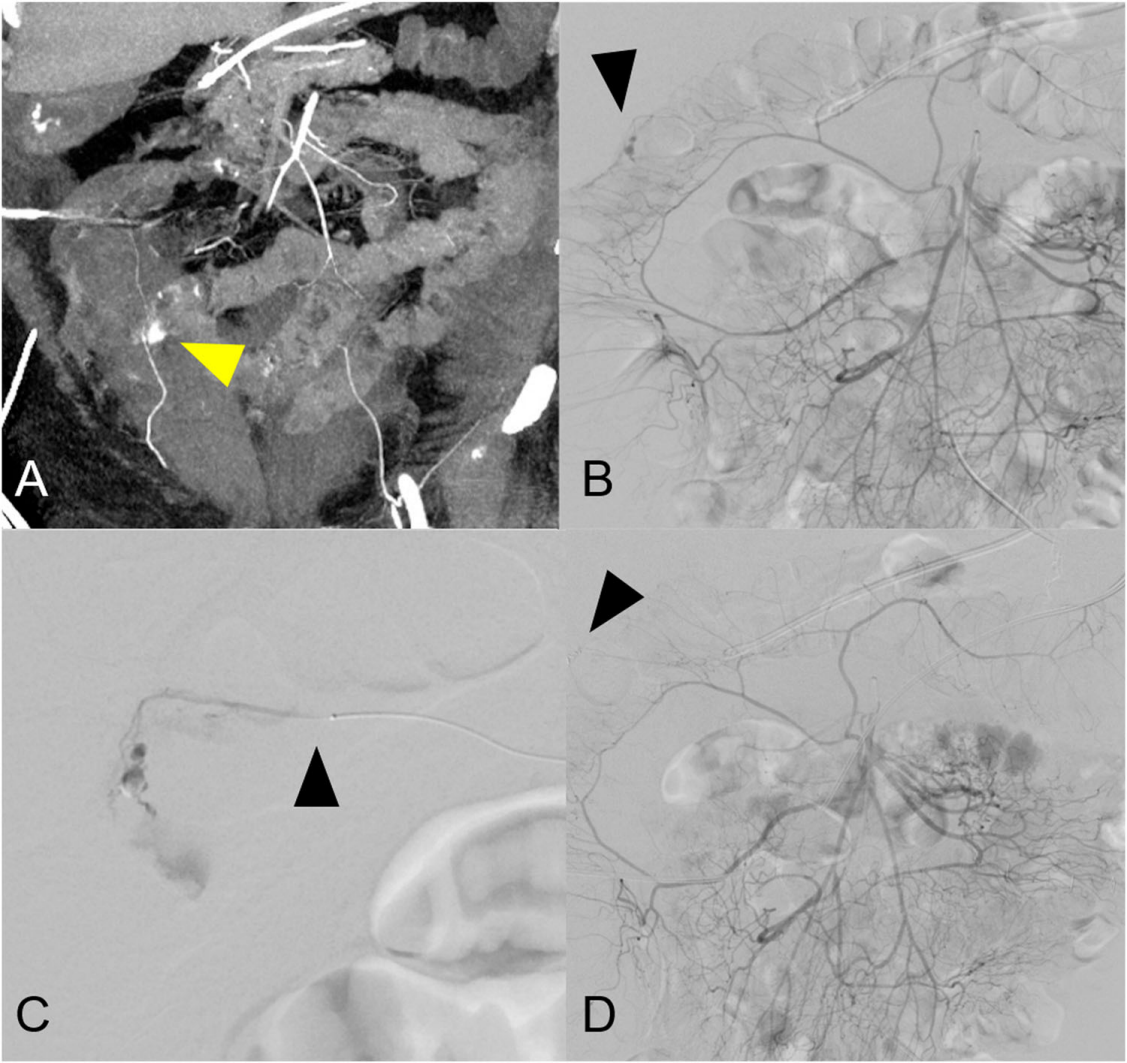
A Contrast-enhanced CT in the arterial phase and coronary slice of a patient with massive rectal bleeding following heart surgery and anticoagulation, where endoscopy revealed no abnormalities. The CT scan identified active arterial bleeding in the region of the right colonic flexure (yellow arrowhead). B A superior mesenteric angiography revealing extravasation in the area of the right colonic flexure (black arrowhead). C A micro-wire and catheter (black arrowhead) were used to navigate into the distal arterial arcade, allowing the bleeding to be successfully treated through coil embolization. D The final mesenteric angiogram confirms that the entire remaining bowel is still adequately perfused while the target vessel was closed with a coil (black arrowhead)
